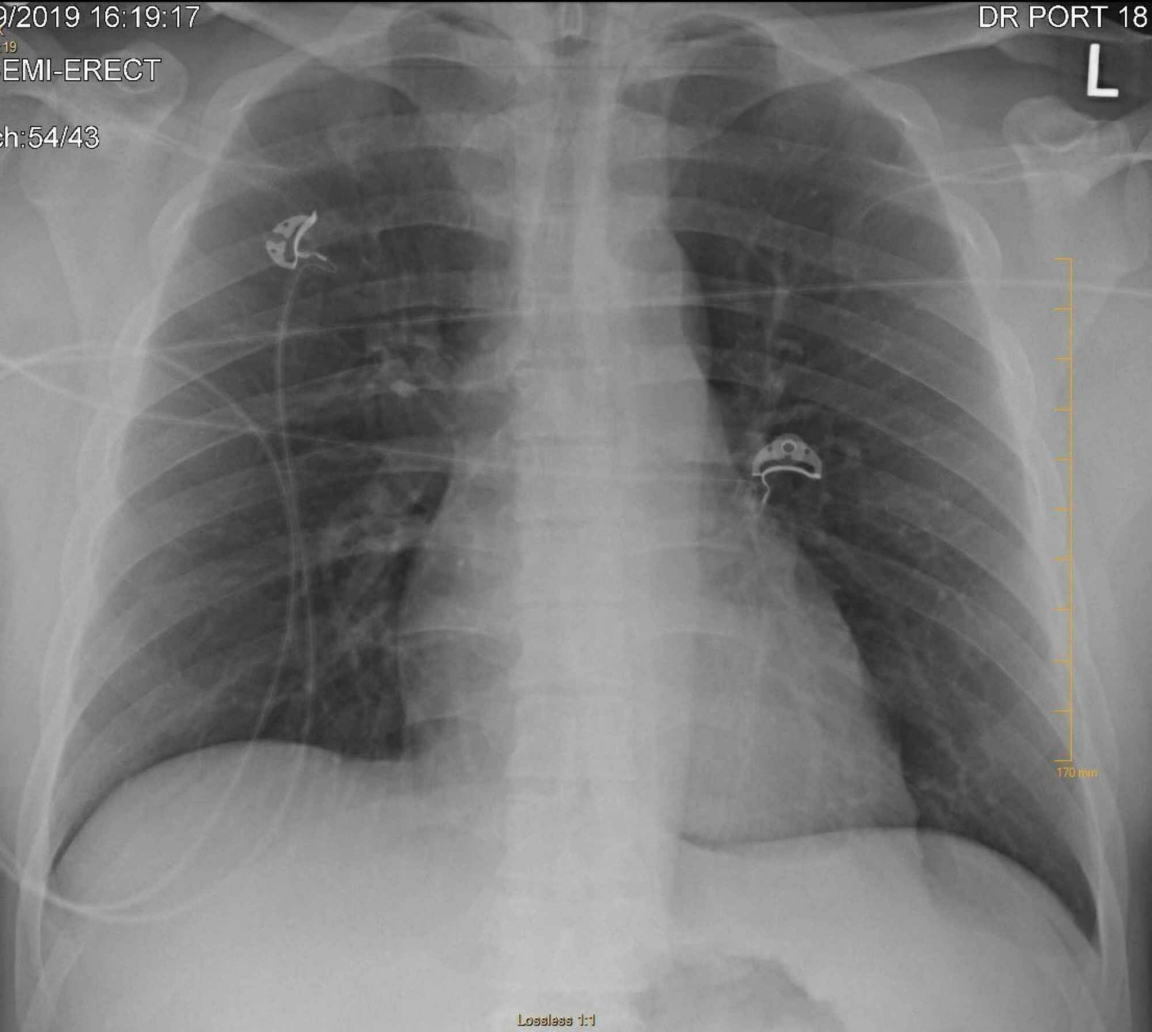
Chest X-ray showing no acute abnormalities.
